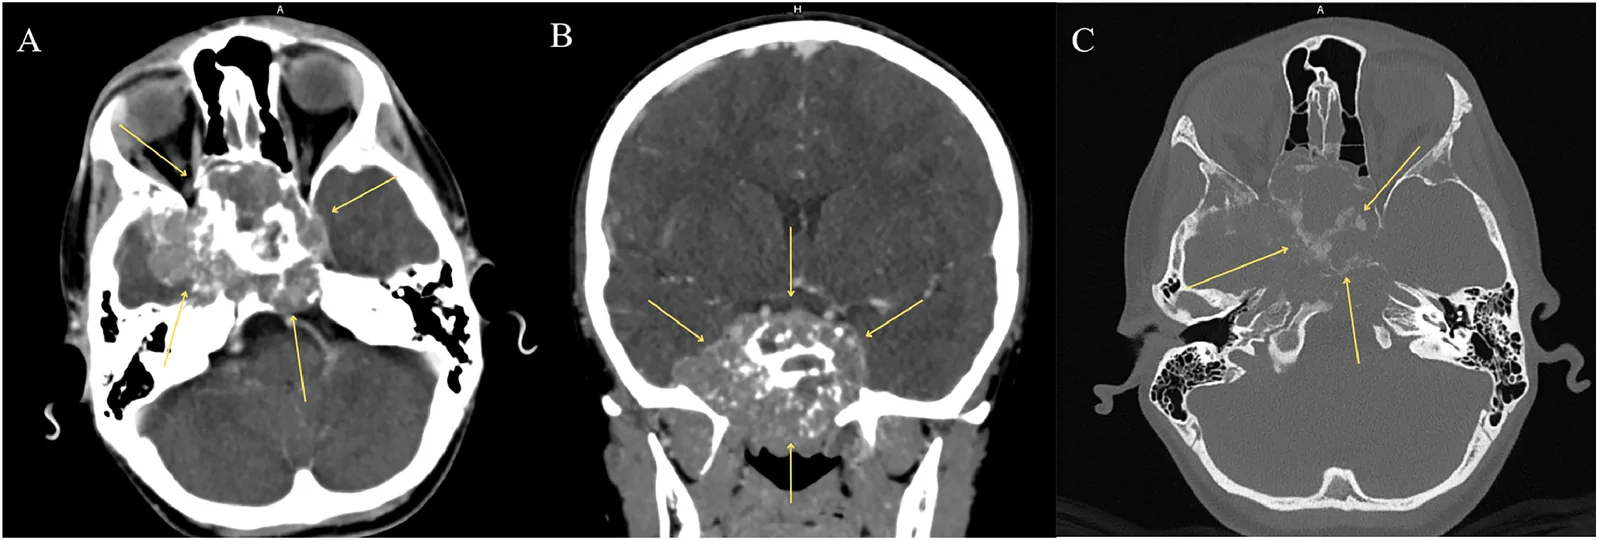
Contrast-enhanced CT of SMARCB1-deficient sinonasal carcinoma in an 8-year-old patient. (A) Axial and (B) coronal post-contrast soft tissue images demonstrate a lobulated, enhancing central skull base mass (arrows), eccentric to the right. Internal hyperattenuation within the lesion corresponds to areas of calcification. (C) Axial bone window image better demonstrates prominent sheet-like calcifications within the lesion (arrows).
